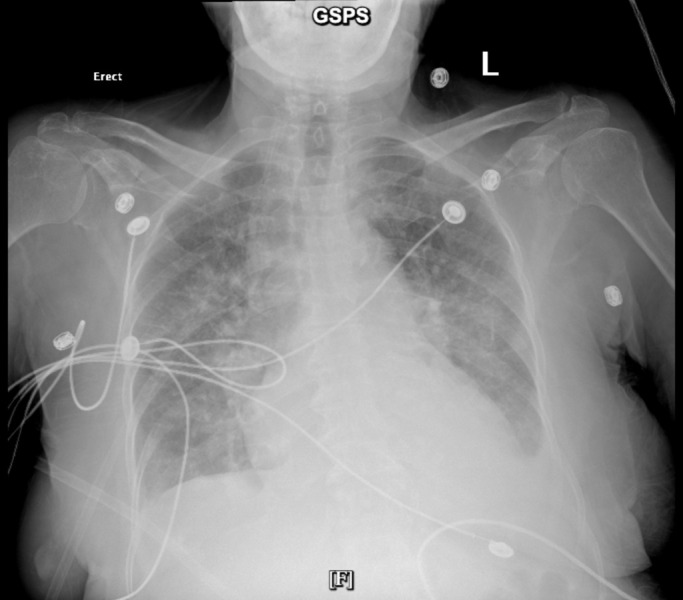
Baseline Chest Xray at initiation of acetazolamide. Portable AP chest radiograph (08/08/2025): Diffuse bilateral perihilar opacities and small pleural effusions consistent with pulmonary edema; cardiomegaly present. Image obtained on hospital day 0, immediately prior to initiation of adjunctive acetazolamide.
